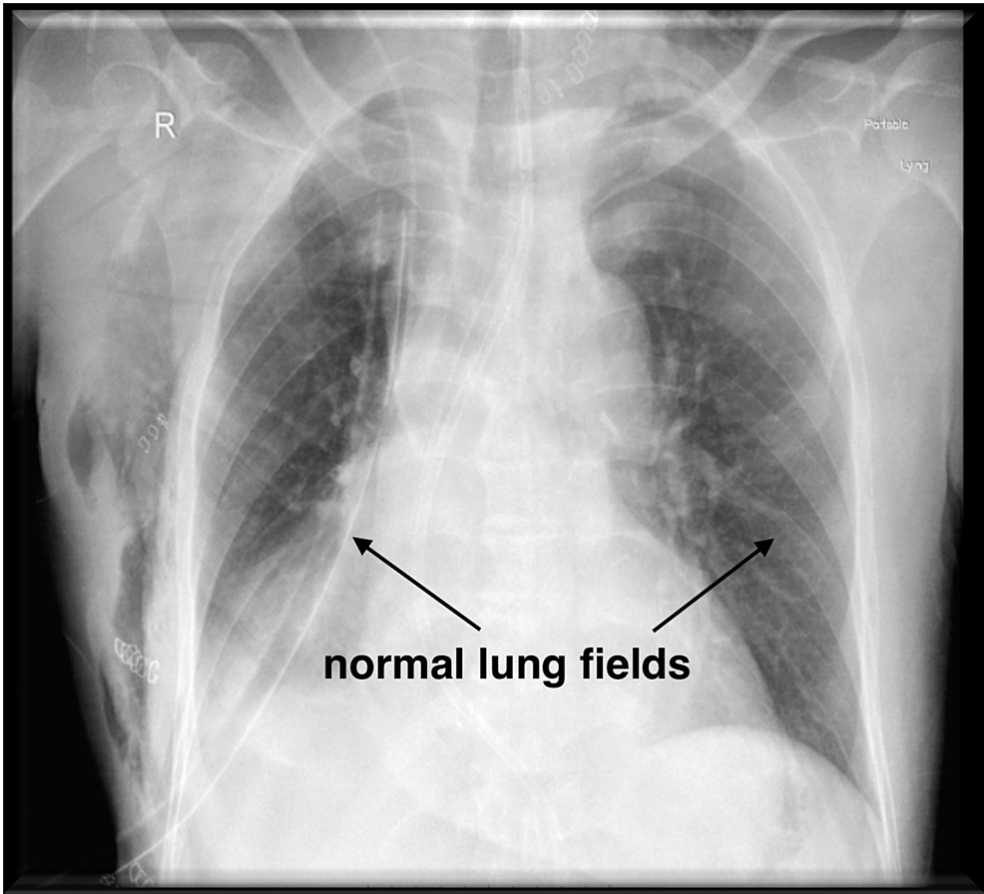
Normal chest x-ray after extubation
